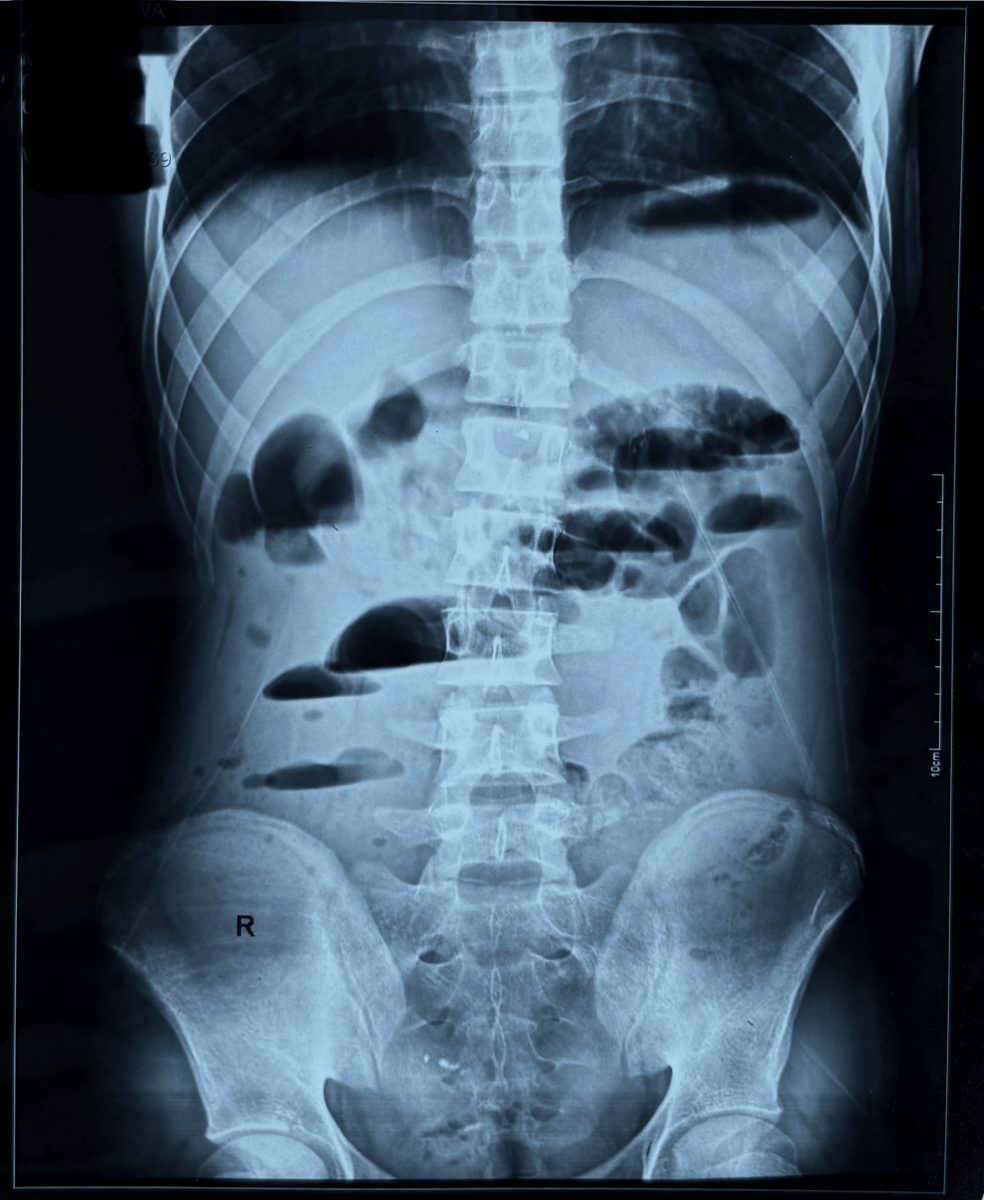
Abdominal radiograph showed dilated intestinal loops and multiple air-fluid levels.
